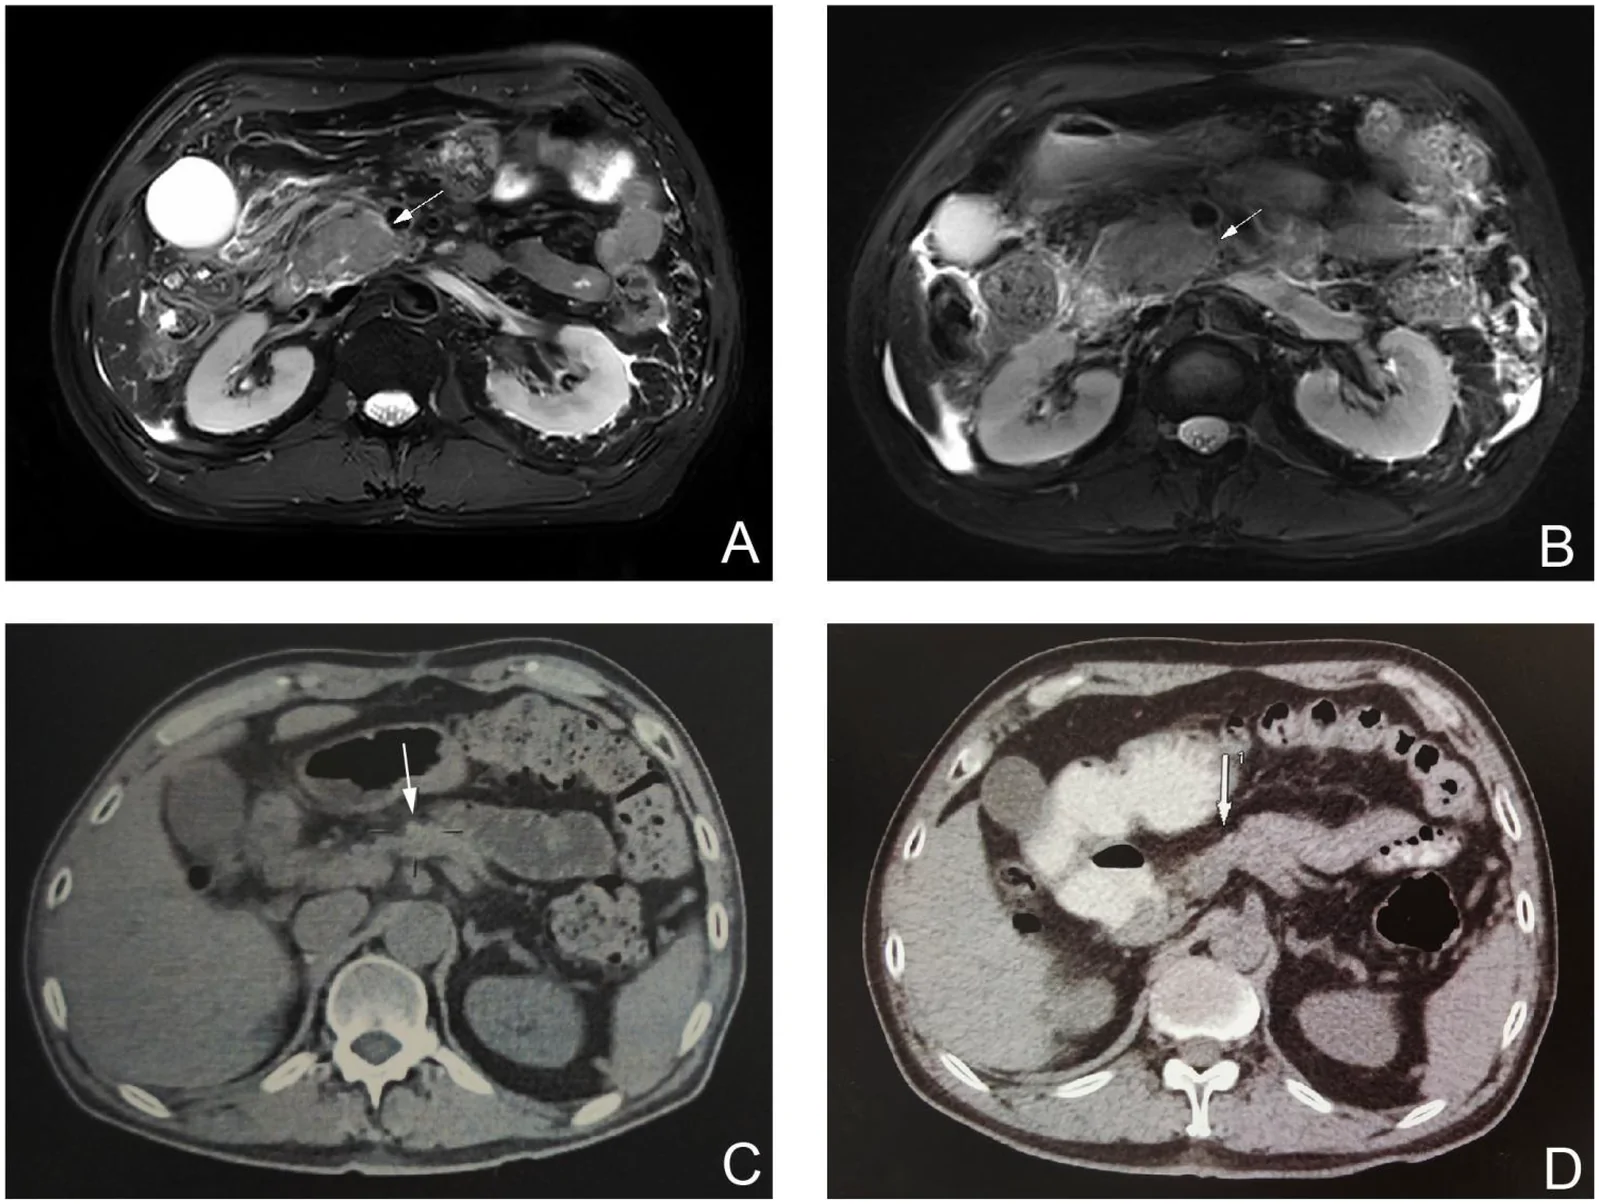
Serial abdominal imaging during treatment. (A) Baseline MRI obtained on February 21, 2024, showed a visible lesion involving the pancreatic head–neck region. (B) MRI obtained on March 21, 2024, after exploratory chemotherapy combined with immunotherapy showed no evident reduction in tumor size. (C) PET/CT obtained on July 27, 2024, showed marked regression of the pancreatic mass to approximately 0.7 cm with an SUVmax of 2.3, accompanied by substantial regression of portal vein tumor thrombosis and metastatic lymph nodes. (D) FAPI PET/CT obtained in October 2024 showed a residual lesion in the pancreatic neck region. White arrows indicate the target lesion.
